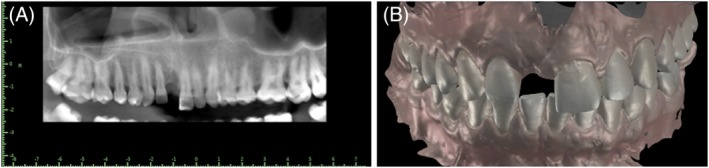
(A) Cone beam computed tomography (DICOM viewer); (B) PLY initial situation.
